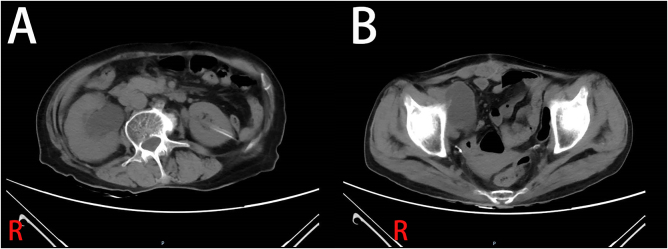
Preoperative CT imaging. (A) Axial view demonstrates significant right-sided hydronephrosis with marked dilation of the renal pelvis and proximal ureter. (B) Coronal view shows the relationship between the ileal conduit and the right pubic bone.
